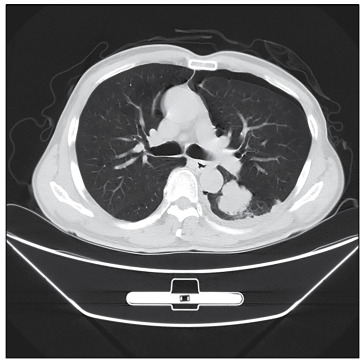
Computed tomography scan showing a mass in lung window.
